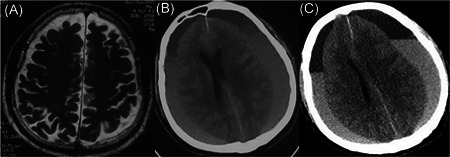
Preoperative head imaging. (A) Before admission, previous magnetic resonance imaging showed bilateral chronic subdural effusion; (B) on admission, computed tomography (CT) showed bilateral chronic subdural hematoma with effusion; (C) 1 day after admission, CT showed that subdural hematoma had increased
